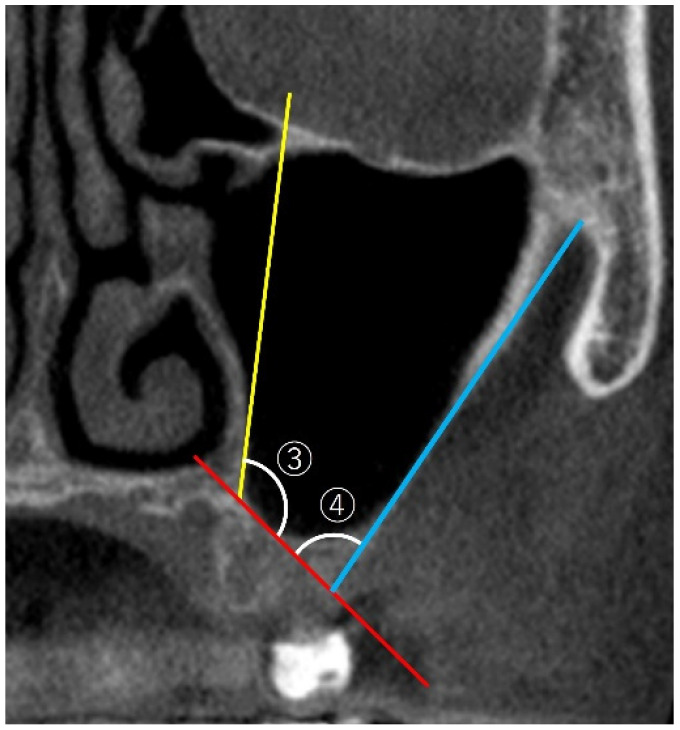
A coronal view of the cone-beam computed tomographic image. ③ PNR angle: the angle formed by two imaginary lines—one along the lower part of the lateral nasal wall (yellow line) and the other along the palatal wall of the maxillary sinus (red line)—was measured at the level of the first molar. ④ MSA: the angle formed by two imaginary lines—one along the lateral wall (blue line) and the other along the medial wall (red line) of the maxillary sinus—was measured at the level of the maxillary first molar.
